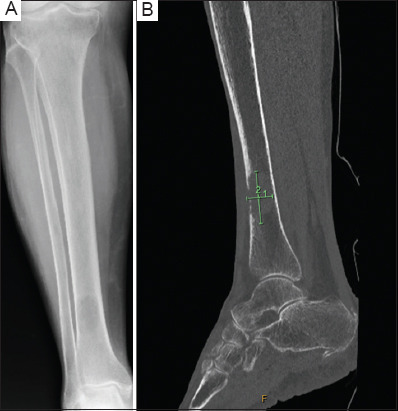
A lesion in the distal tibia of the right leg. (A) X-ray scan of the right lower leg (anterior-posterior view) showing a lesion in the distal tibia. (B) Non-contrast computed tomography scan of the right lower leg (sagittal view) confirms a lesion of 41 × 20 mm with a permeative breach of the anterior cortex
